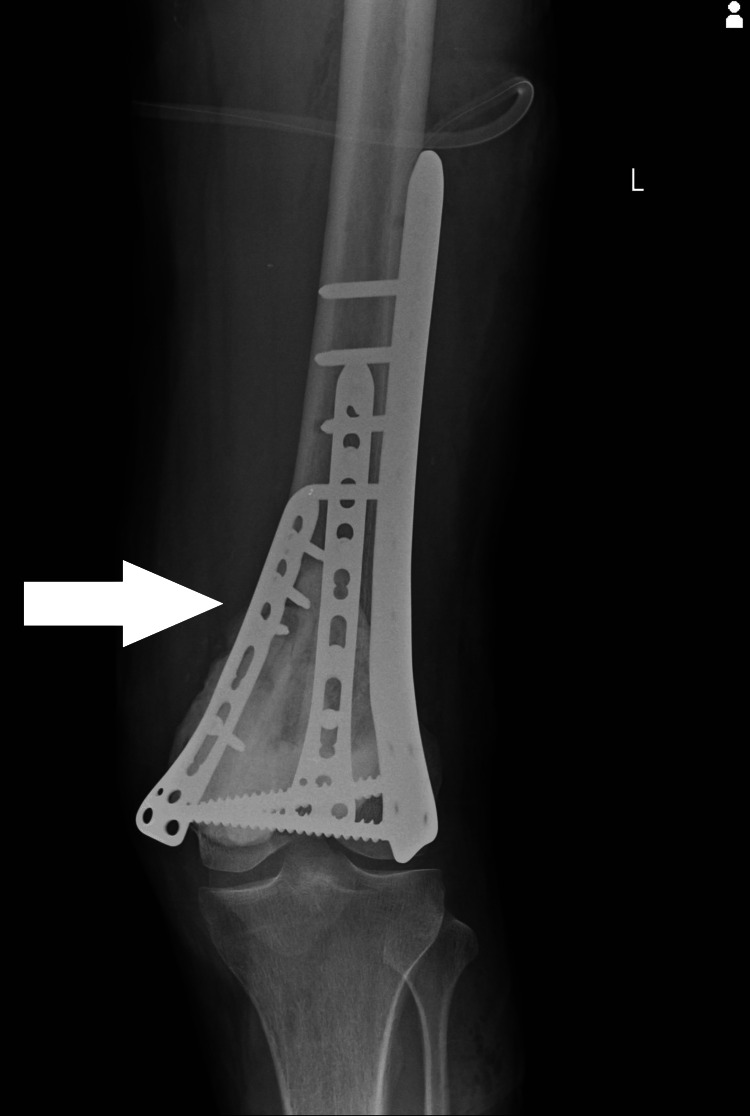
X-ray image depicting locking plate used for bone grafting
